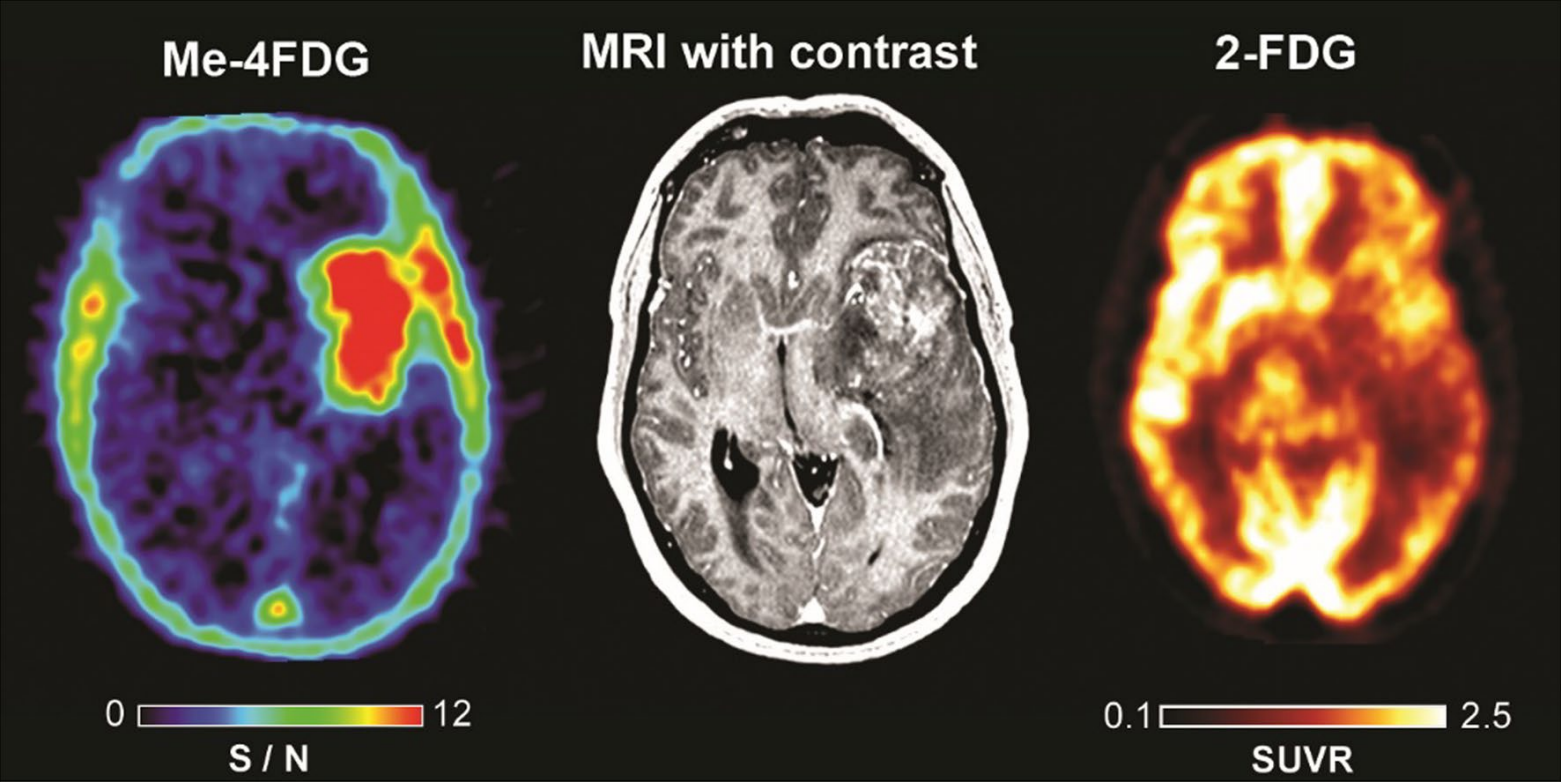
Me-4FDG PET, 2-FDG PET and MRI scans on a patient with an anaplastic astrocytoma (WHO Grade III). The Me-4FDG S/N (SUVR/BG) scales are for the NIH color scale and the 2-FDG SUVR scale is for the “Hot-Iron” color scale
